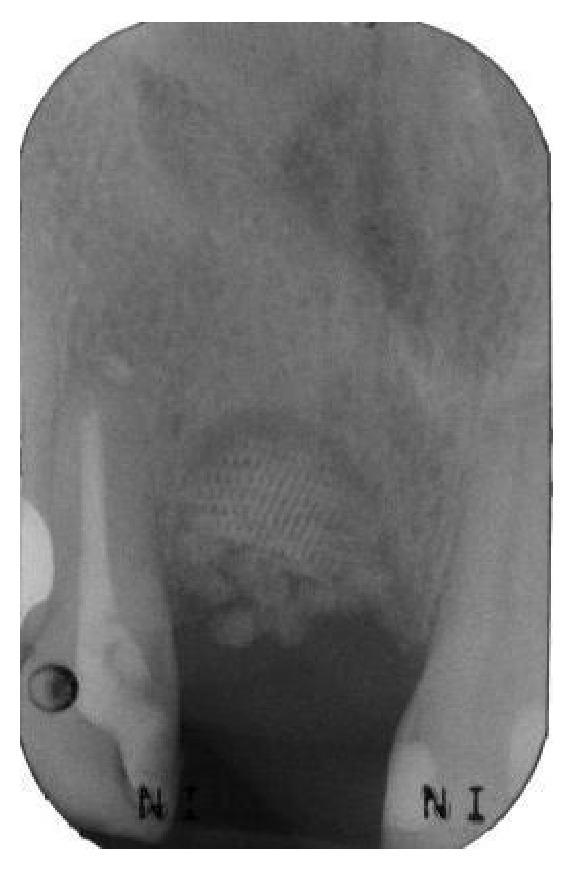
After 6 months of uneventful healing, a periapical radiograph showed good integration of the material used for regeneration.
